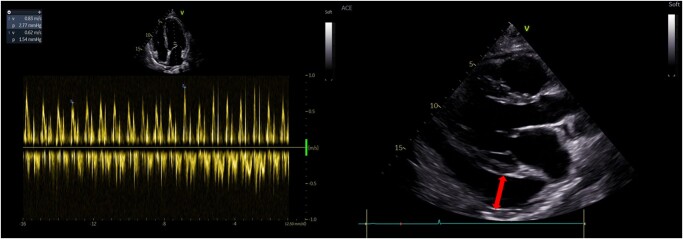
‘Swinging heart’ and 25% breathing variability of mitral pulsed Doppler.
